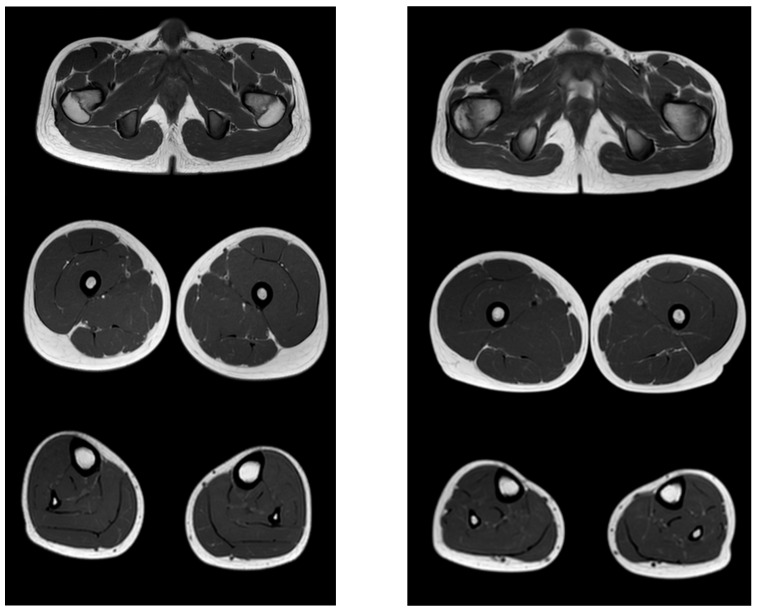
MR images of healthy volunteers 13 years old (left) and 8.3 years old (right). From top to bottom: the pelvic level, the femoral level and the level of the legs.
